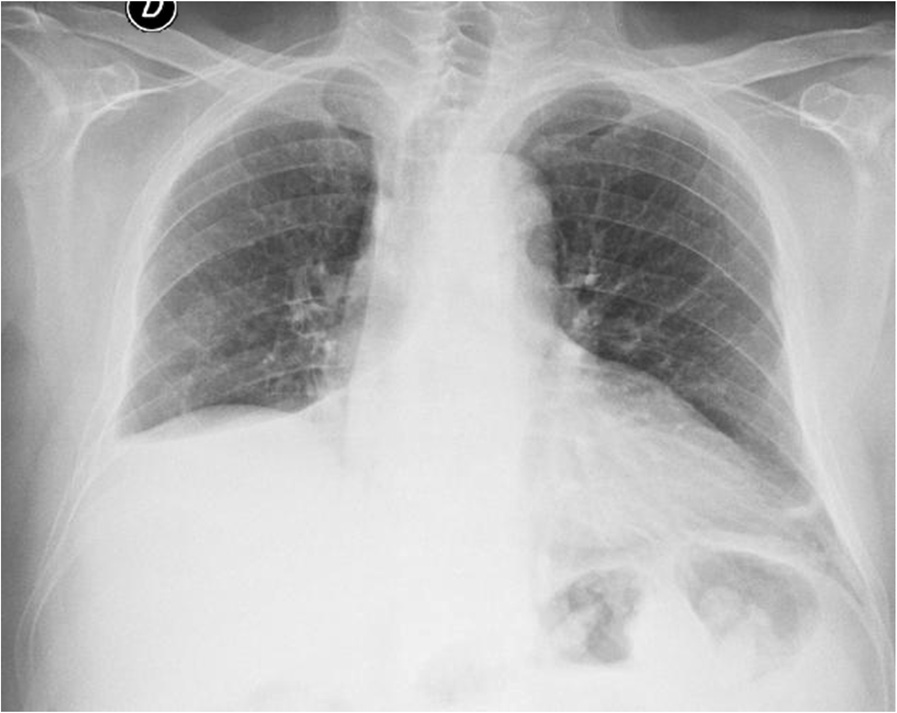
Frontal chest radiograph showing right lower lobe collapse.
